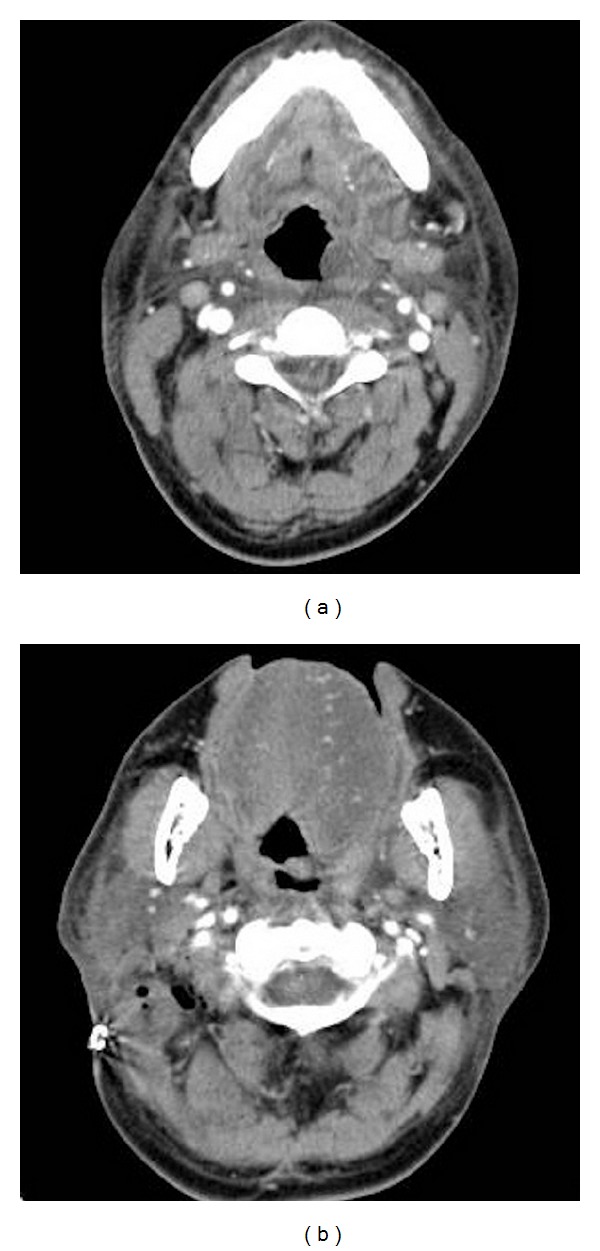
Postoperative contrast-enhanced computed tomography of the neck. Sixteen hours after the operation, swelling of the neck, tongue, pharynx, and vocal cords was observed on the left side.
